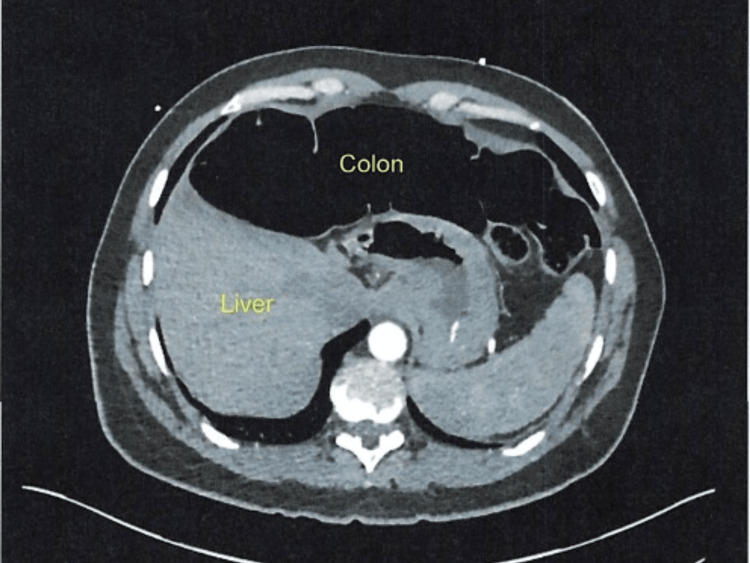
CT of the abdomen revealed a hyperlucent appearance involving the diaphragm diffusely with a lobular appearance, consistent with bowel gas and Chilaiditi syndrome.
